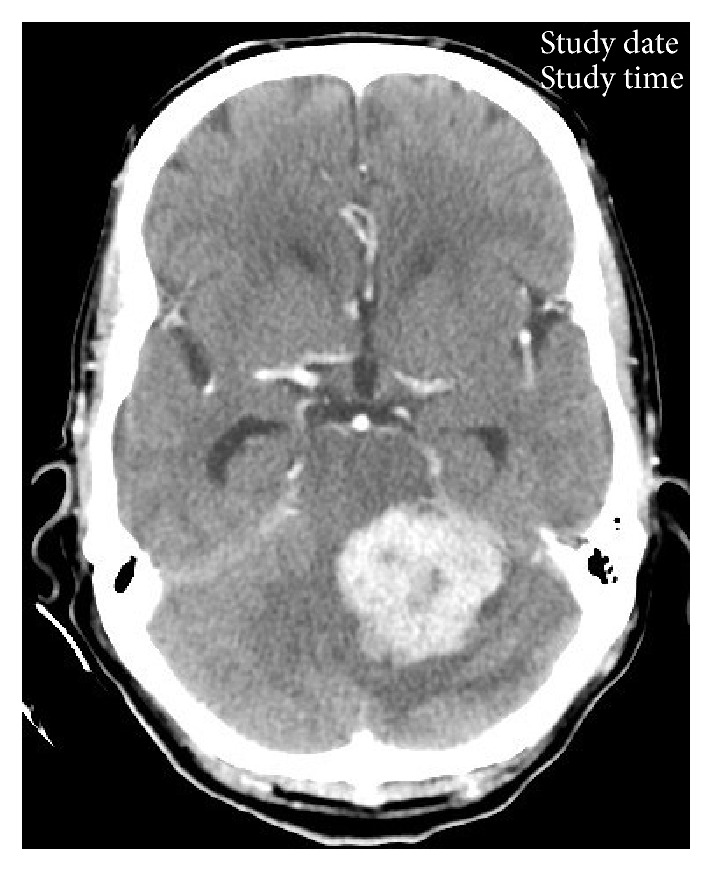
Computed tomography of brain (axial view) showing a 3.2 × 3.7 cm homogeneous contrast enhancing mass in the inferior vermis with extension into the left cerebellar hemisphere and brainstem causing hydrocephalus (the second case).
